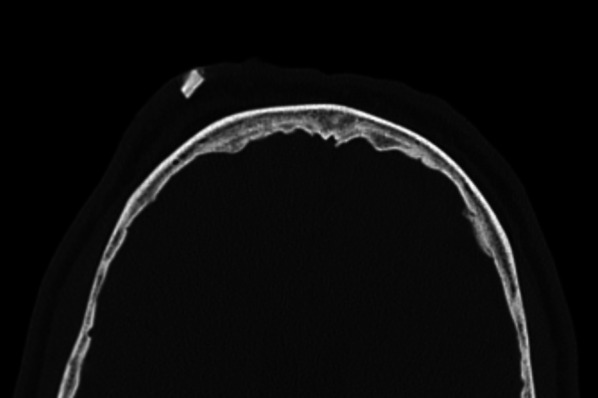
Stone. Axial CT showing a hyperdense structure in the subcutaneous tissues in the right frontal area
